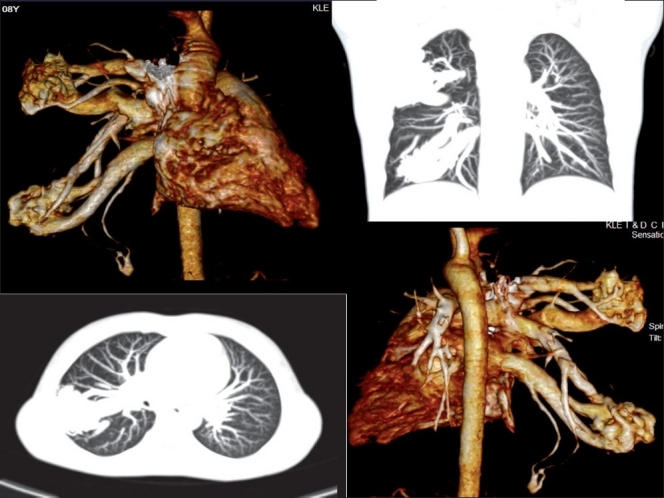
CT pulmonary angiogram showing multiple pulmonary arterio venous malformations
